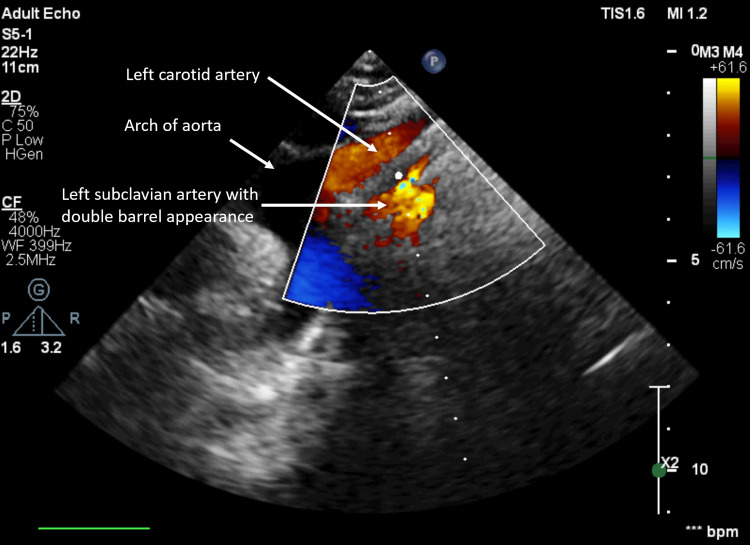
Suprasternal view with color Doppler showing the aortic arch and its main branches.
